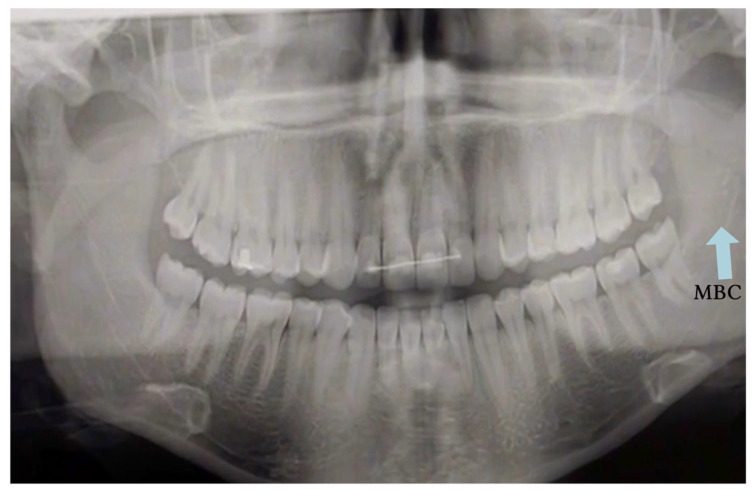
Panoramic radiograph (PR) showing a suspected bifid mandibular canal (BMC; blue arrow). The blue arrow indicates the cortical outline suggestive of canal duplication. PR alone cannot confirm this finding; CBCT is recommended when confirmation would change management (CBCT not shown).
